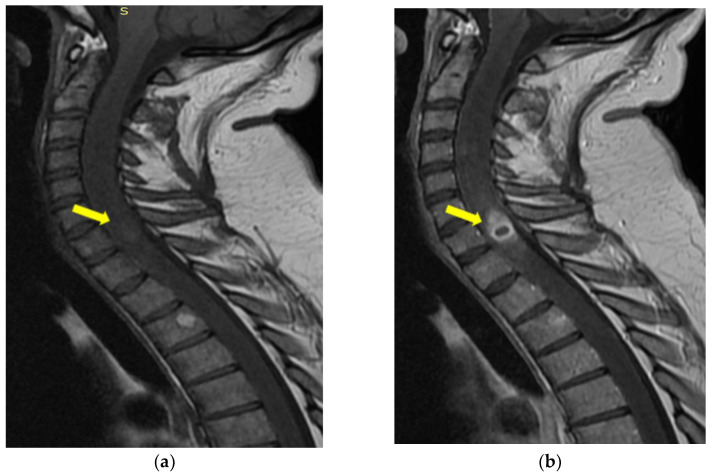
MRI of the cervical sagittal spine T1-weighted image (a) and after intravenous Gd-chelates (b): ring-enhancing mass within cord. The yellow arrow indicates the expansive cystic intramedullary process.
